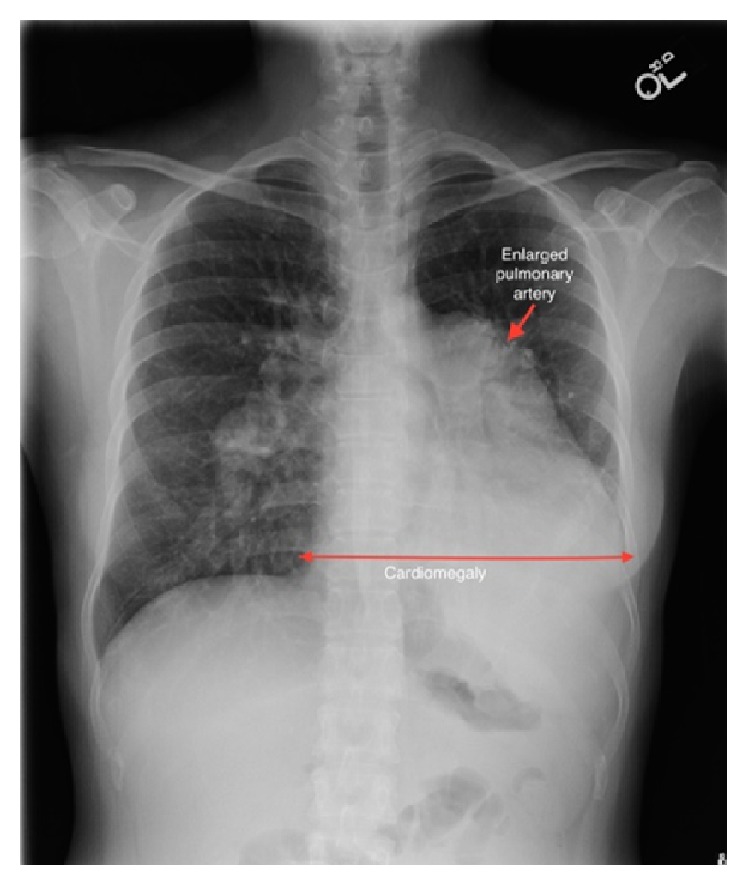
A chest radiograph shows a markedly enlarged pulmonary trunk (red arrow), enlarged central pulmonary arteries, and enlargement of the cardiac silhouette.
